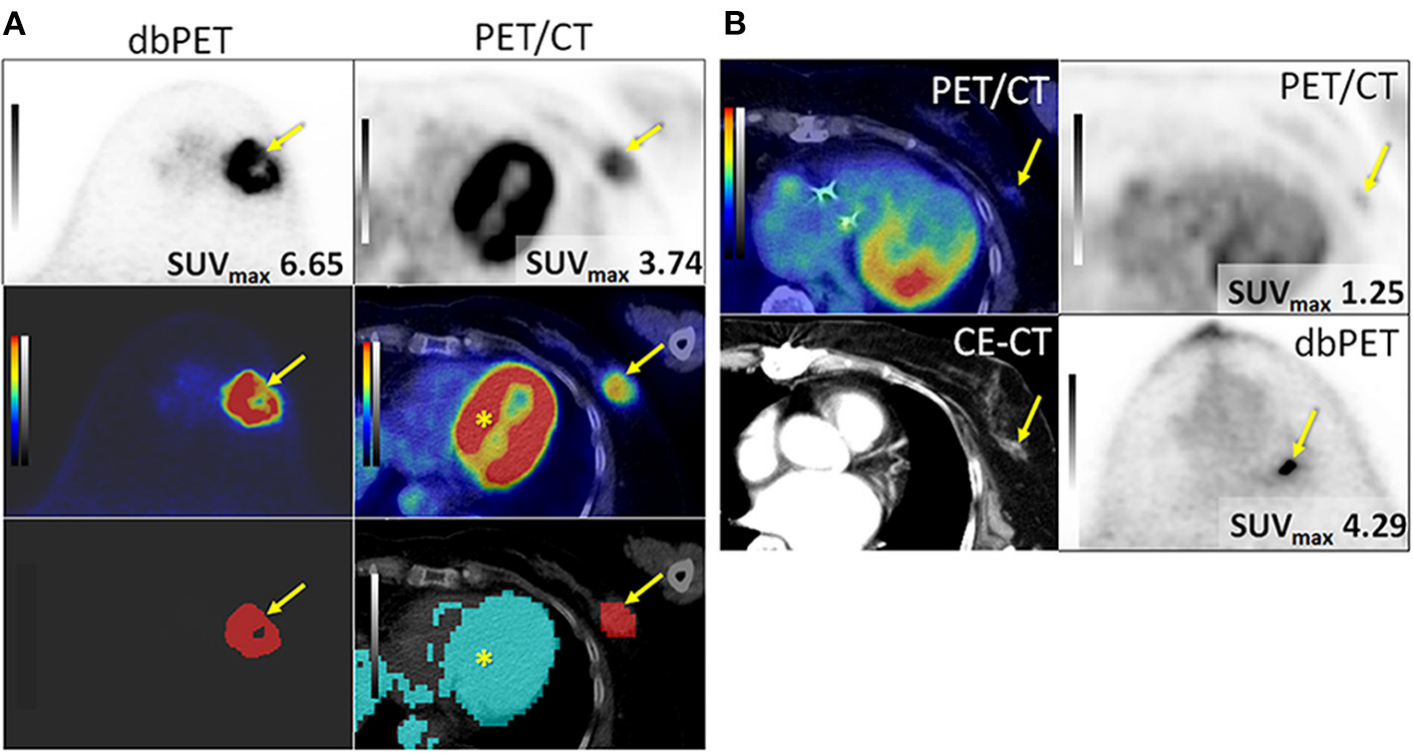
Successfully (A) and unsuccessfully (B) extracted breast cancers with dbPET and whole-body PET/CT. (A) Invasive ductal carcinoma in the left breast of an 84-year-old woman. BC (arrow) was successfully extracted with both dbPET and whole-body PET/CT (two images at the bottom) and clearly separated from the physiological uptake of the myocardium (*) with whole-body PET/CT. (B) Invasive ductal carcinoma in the left breast of a 74-year-old woman. It was successfully extracted with dbPET but failed with whole-body PET/CT; therefore, it was excluded from this study. BC, breast cancer; CE-CT, contrast-enhanced computed tomography; dbPET, dedicated breast positron emission tomography.
